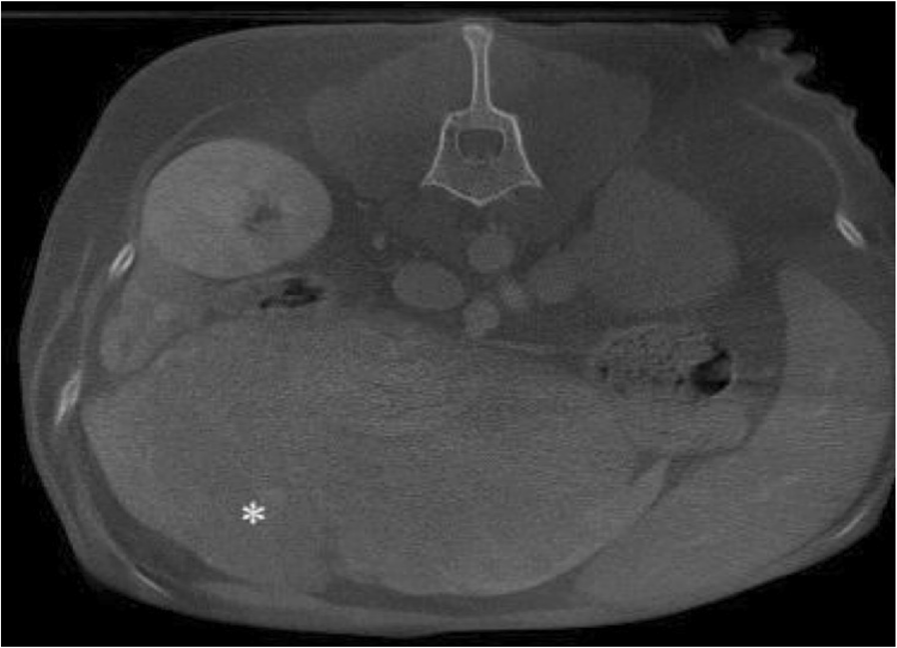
Transverse reconstructed postcontrast, soft tissue algorithm, computed tomography image of the abdomen. Attenuating mass of the left lateral liver lobe (*)
